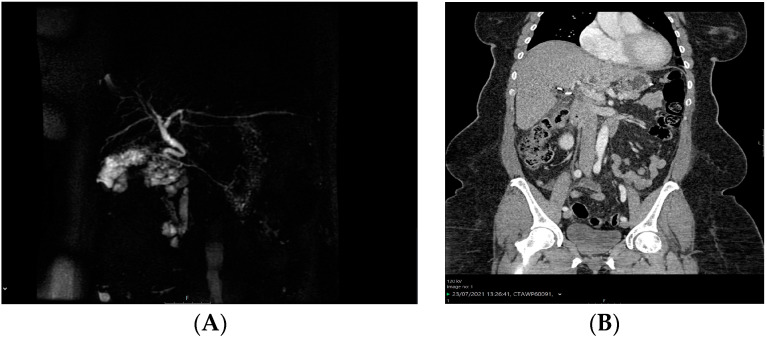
Imaging work-up on postoperative day 1 after the rise of the LFTs and bilirubin levels. (A) MRCP after laparoscopic cholecystectomy and (B) abdominal CT scan after laparoscopic cholecystectomy. No injuries or other intra-abdominal complications are present.
